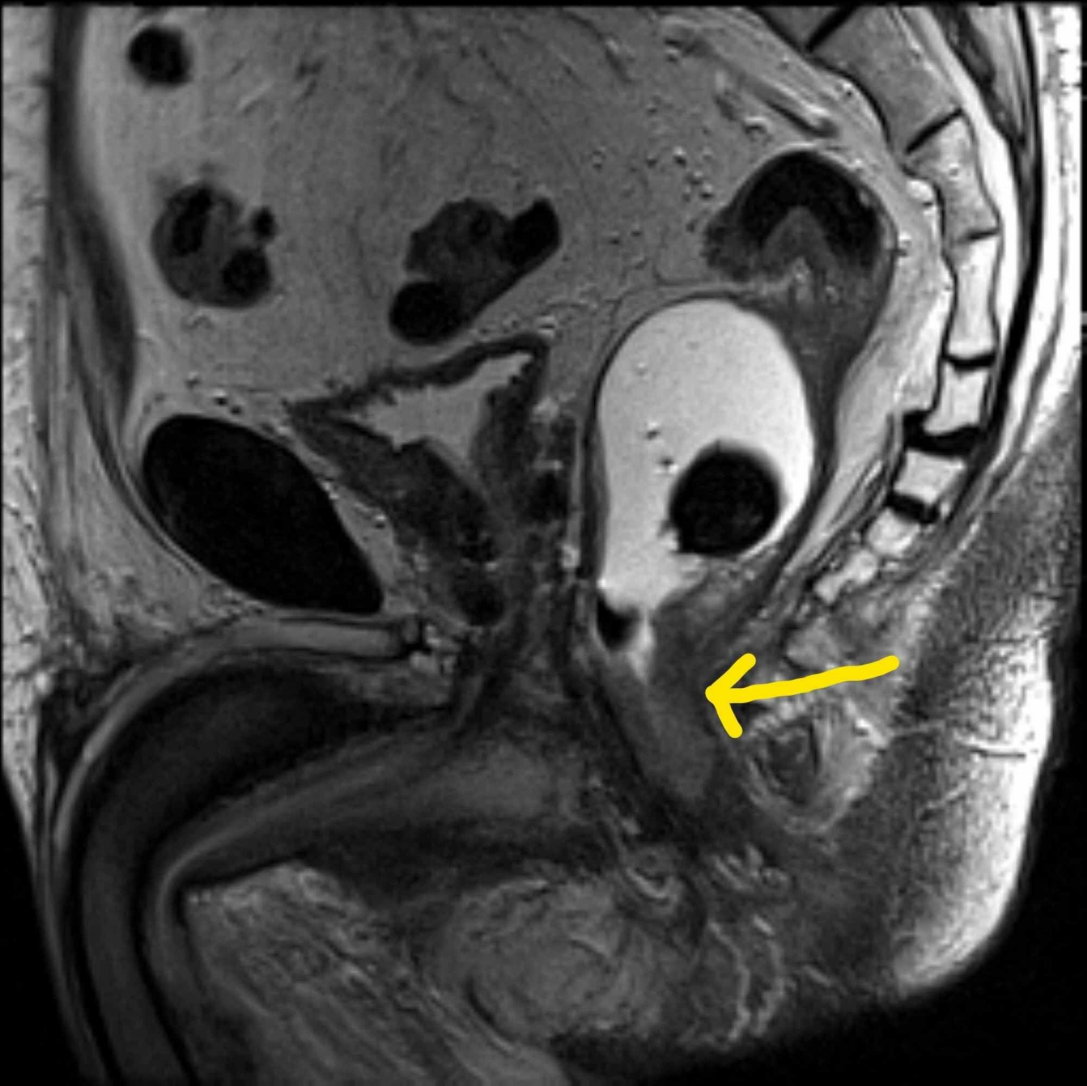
Pelvic MRI showing rectal mass
